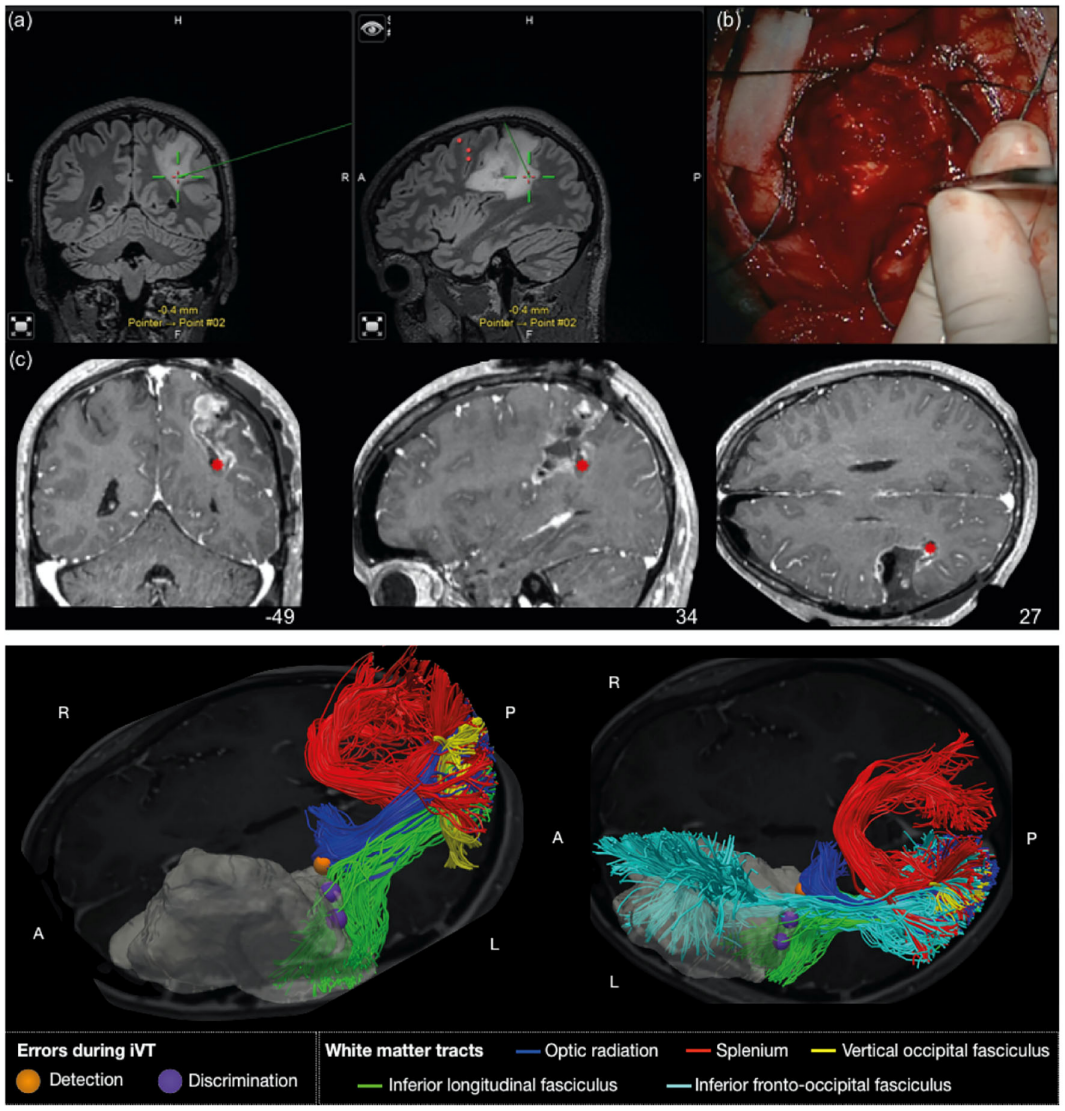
Representative examples of glioma cases in which the iVT was performed intraoperatively for OR detection and preservation. A case of presumptive right parietal lower-grade glioma. This young male patient had a recent clinical history of sensory-motor seizures. A right tumor mass involving the parietal lobe was documented at the MR study; representative (a) coronal—left panel and sagittal—right panel. FLAIR Images, taken as a snapshot during surgery (the red dots indicate the cortical sites corresponding to M1). The patient was operated on in asleep-awake-asleep anesthesia, and submitted during the awake phase to subcortical language, haptic, cognitive, motor, and visual (iVT) mapping. While the patient was performing iVT several sites interfering with the task execution during subcortical DES (LF, 4 mA) were identified. A site where DES induced a detection error is shown (b) in the intraoperative picture on the operative field and tracked with the neuronavigation probe. The location is indicated in the intraoperative snap shots (a) as a green arrow. The location of this site was also reported, co-registered, and superimposed on the post-operative postGD-T1 weighted MR (c), coronal, sagittal and axial images, as a red dot. Histo-molecular diagnosis documented a grade III IDH1 mutated, MGMT methylated astrocytoma. A case of right fronto-temporo-insular presumptive lower-grade glioma, in a 35-year-old male patient. The patient was operated on in asleep-awake-asleep anesthesia, and submitted to motor, cognitive, haptic, motor, and visual (iVT) mapping. The tumor volume is represented in gray in the brain3D reconstruction along with reconstructed tracts (HARDI) surrounding and/or infiltrating the tumor mass: on the left is the axial view from above; on the right is the axial view from below; P, posterior; A, anterior; L, left; R, right. The sites giving interferences while stimulated during iVT task (LF 3 mA) are superimposed to the map as dots. Detection errors are in orange, while discrimination is in purple. Detection errors correspond to OR; discrimination to ILF. The names of the reconstructed tracts are reported in the legend.
